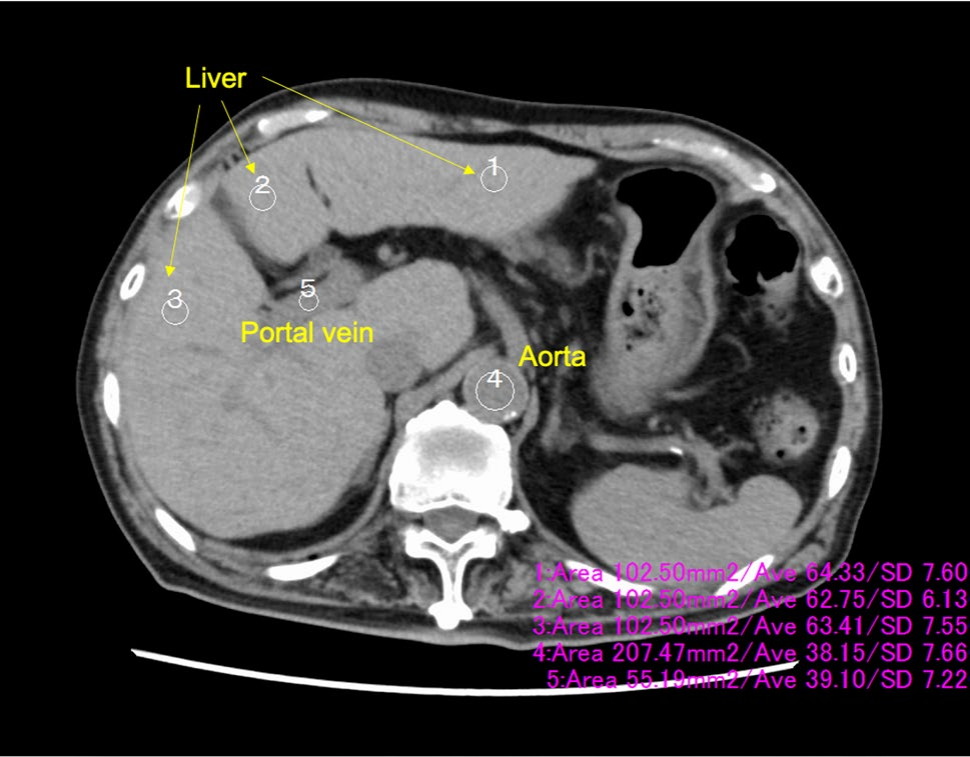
The region of interest was placed in a portal vein inflow slice. The CT values and standard deviation were measured in the liver, aorta, and portal vein
